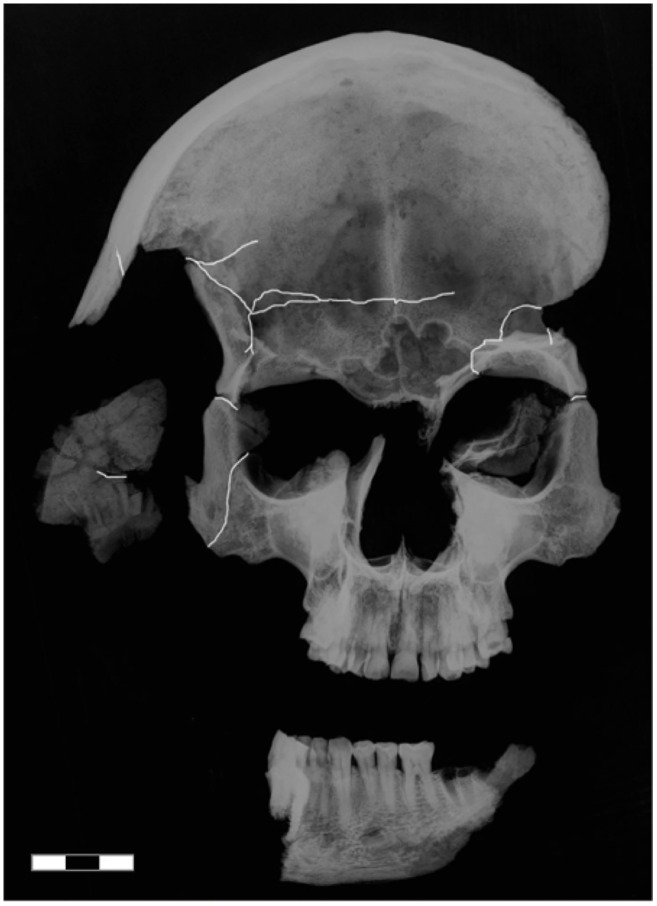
Anteroposterior radiograph of the skull. Fracture lines are emphasized with white lines. Scale bar 3 cm.
